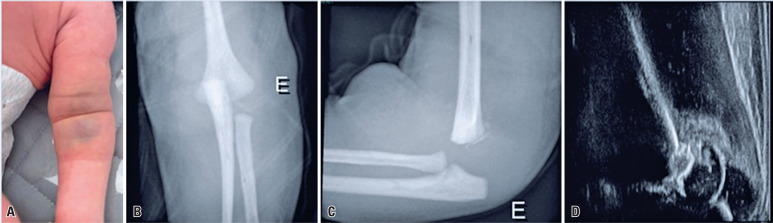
A) Clinical aspect of the left elbow; B and C) X-rays in the anterior–posterior and lateral view; D) ultrasound of the left elbow
